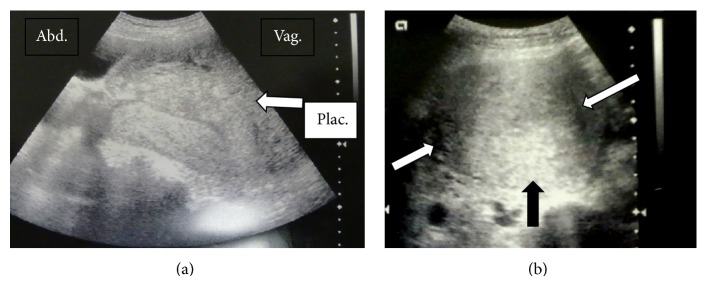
(a) Transabdominal sagittal sonogram showing a mirror image of the uterus with the endometrial pseudostripe represented by the two opposing serosal surfaces [5]. The placenta that adhered to the fundus can be seen on the vaginal side (white arrow). (b) Transabdominal transverse sonogram showing the target sign with the hyperechoic inverted fundus centrally (black arrow) surrounded by hypoechoic fluid (white arrow) between the fundus and vaginal wall [5].
